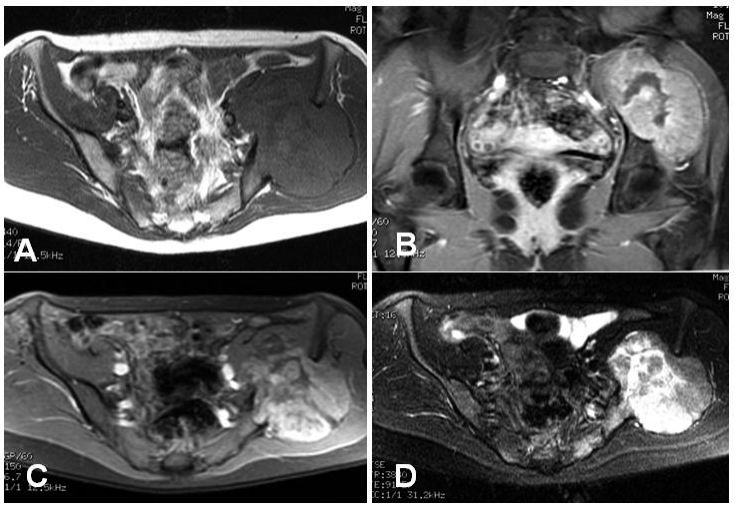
a-d: MRI shows a mass originating from the ileum and extending into the soft tissues both anteromedially and posterolaterally. In T_1_-weighted sequences the mass is isointense with muscle (a). It enhances after administration of gadolinium (b, c). In fat-suppressed T_2_-weighted sequences it has high signal intensity (d).
